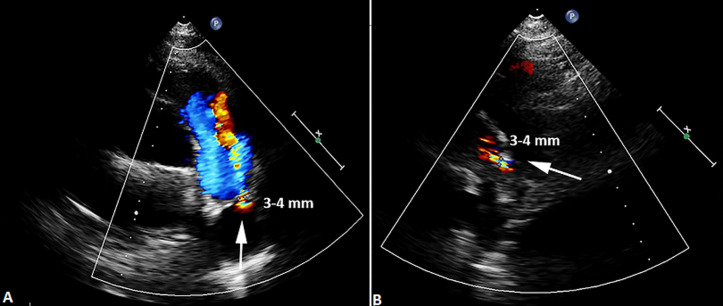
two-dimensional echocardiography showed the small residual PDA
